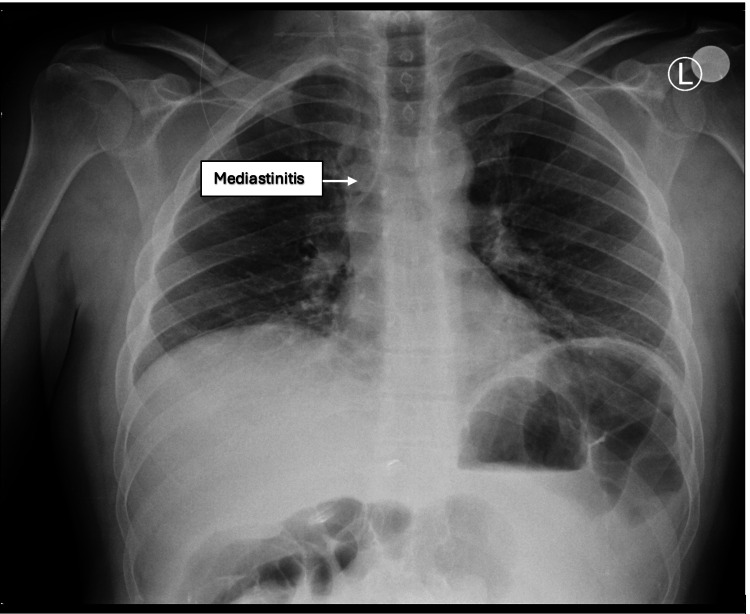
The patient's chest X-ray on admission. The presence of the widening and haziness of the mediastinum as indicated by the white arrow prompted further investigations.
